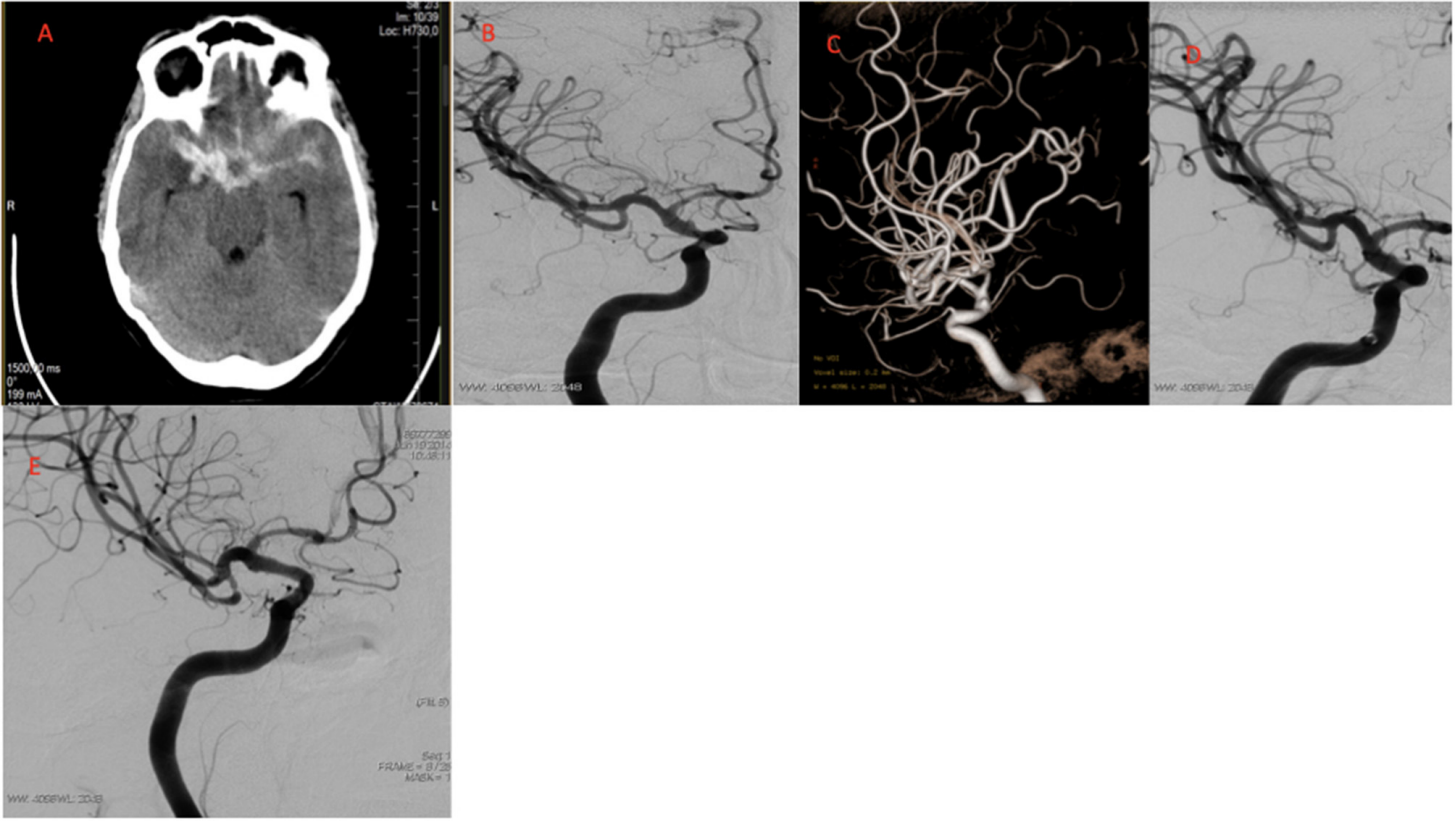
Images of a typical case. (A) CT images after headache showing SAH Fisher CT grade 3. (B) Conventional DSA obtained before treatment showing supraclinoid ICA BBA. (C) 3D angiogram of BBA. (D) Immediate control angiogram OKM C type anurysm filling. (E) 3 month control angiogram OKM D type complete obliteration. CT, computed tomography; SAH, subarachnoid haemorrhage; DSA, Diagnostic cerebral angiography; BBA, Blood blister like aneurysm; OKM, O'Kell–Marotta grading scale.
